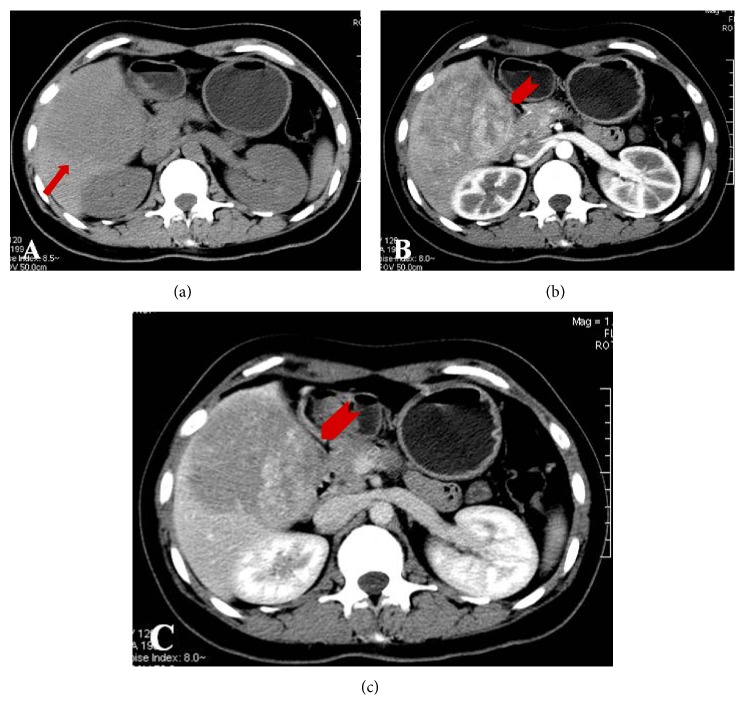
Unenhancement CT (a) images reveals a large well-demarcated hypoattenuate mass in the right lobe of the liver (arrow). No fat tissue is detected at CT images. The mass shows heterogeneously intense enhancement at hepatic arterial phase ((b) arrowhead) and persistent enhancement at portal venous phase ((c) arrowhead).
